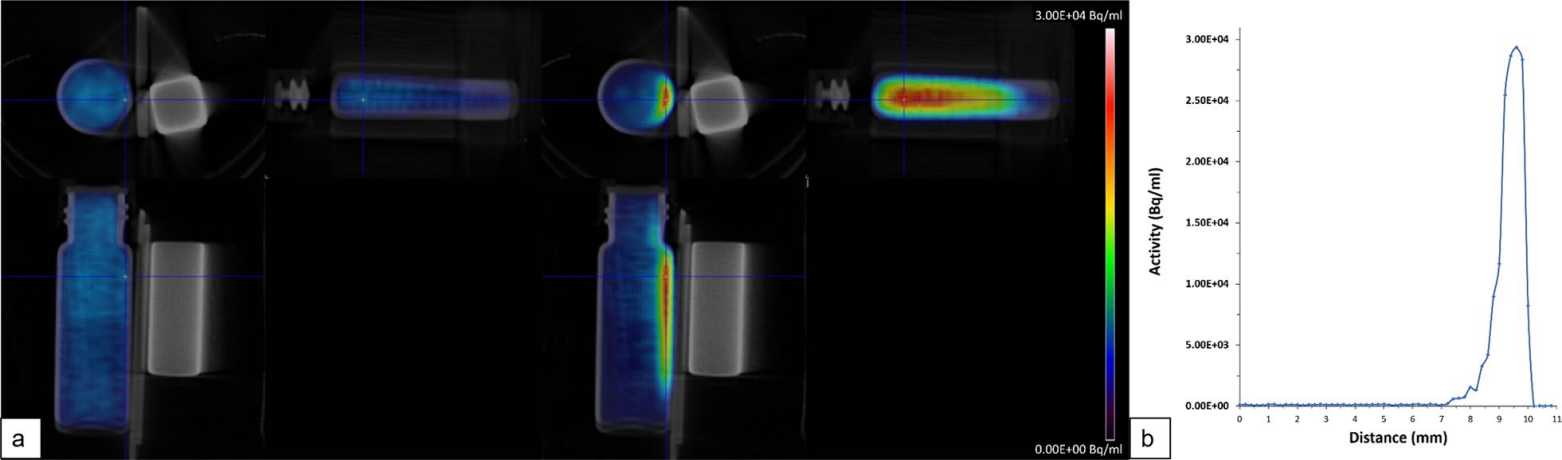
PET/CT images of the distribution of 5.3 MBq activity [^68^Ga]MNPSNP@THP in an ampoule exposed to neodymium magnet at the start of the 30 min dynamic acquisition and at 30 min (a) and central cross-section activity gradient in the vial at 30 min (b)
